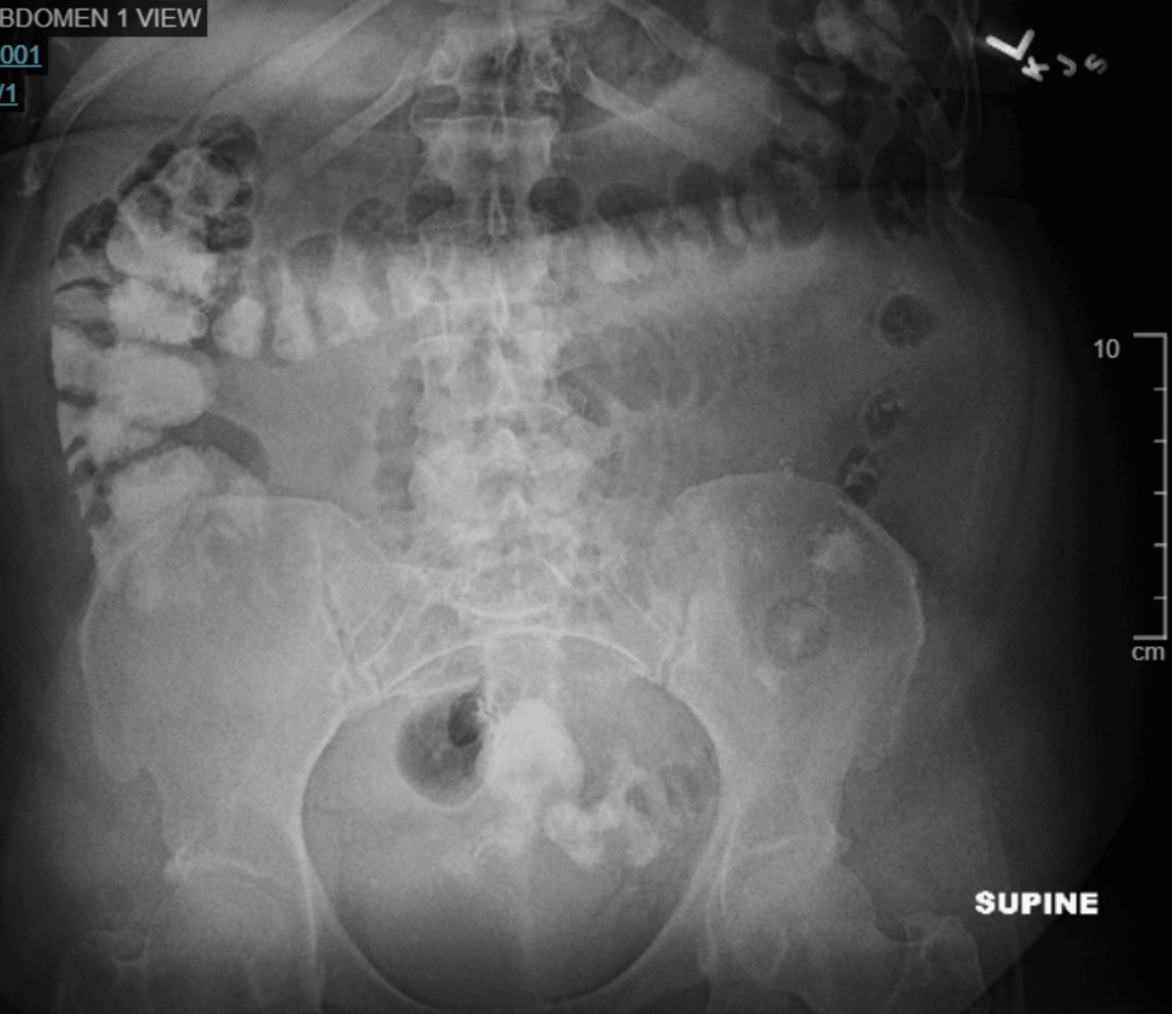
AXR obtained six hours after contrast administration in a patient with SBO AXR, abdominal radiograph; SBO, small bowel obstruction
